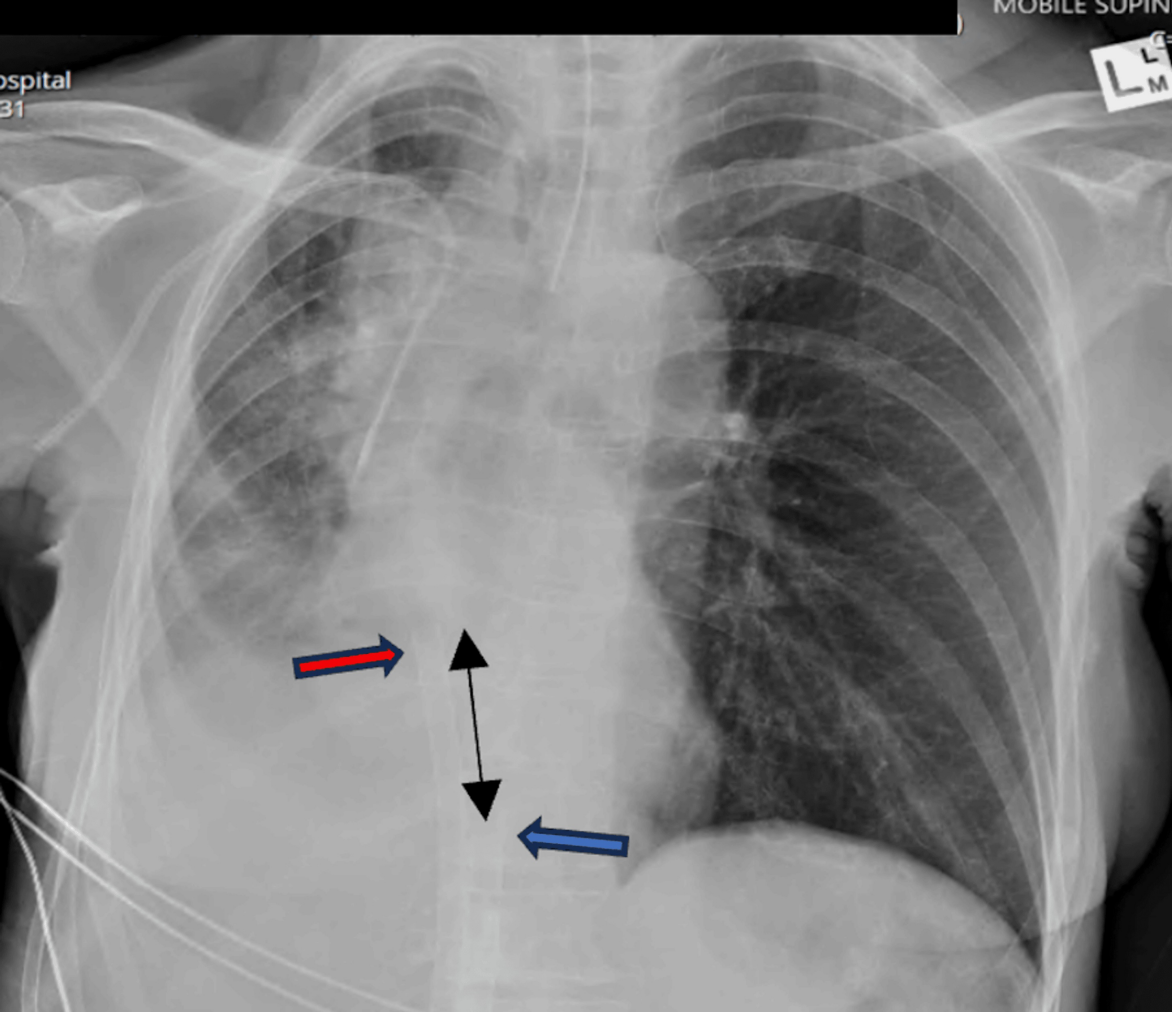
CXR post VVECMO cannulation. Multistage access cannula at the cavo-atrial junction (blue arrow) and single-stage return cannula (red arrow) positioned in the mid-right atrium. CXR, chest X-ray; VVECMO, veno-venous extracorporeal membrane oxygen
